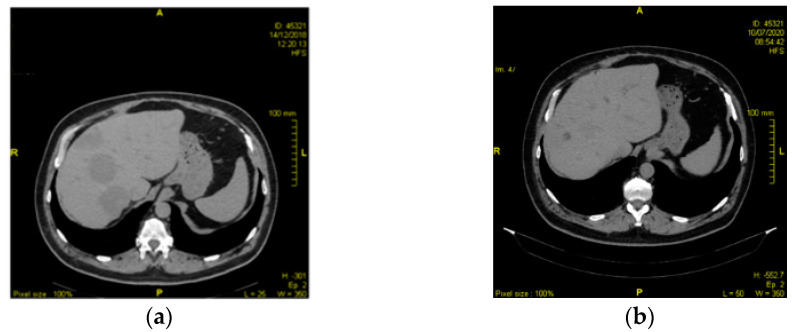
(a) CT scan, March 2019; (b) CT scan, September 2020.
